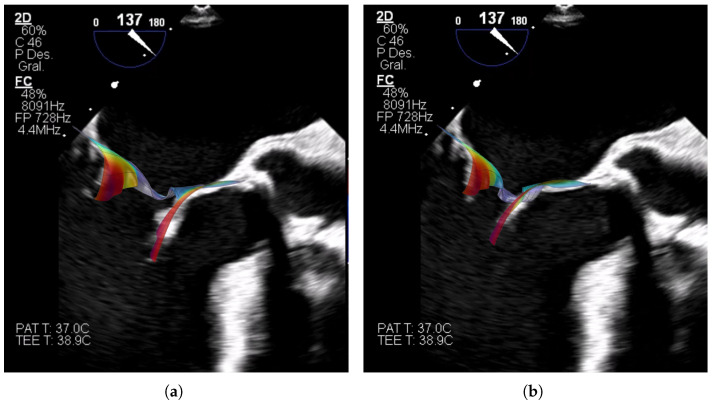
Comparison of the leaflet shape and annulus distance geometry with the ultrasound images at two points during closing. (a) At $t_{0.45}=0.09$ s. (b) At $t_{0.5}=0.1$ s.
